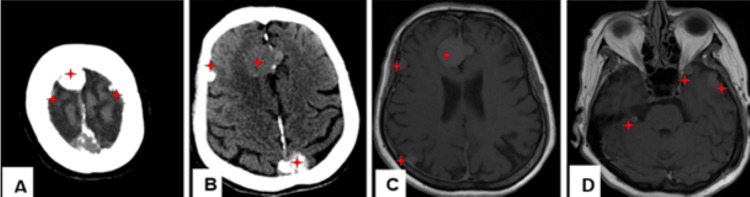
(A) and (B) Axial CT scan, (C) and (D) MRI axial T1W after IV gadolinium contrast administration, showing multiple extra-axial supra- and infratentorial lesions throughout the brain, the majority being calcified, and enhancing after IV gadolinium administration, compatible with mengingiomatosis (red asterisk). CT: computed tomography; MRI: magnetic resonance imaging; T1W: T1-weighted; IV: intravenous
